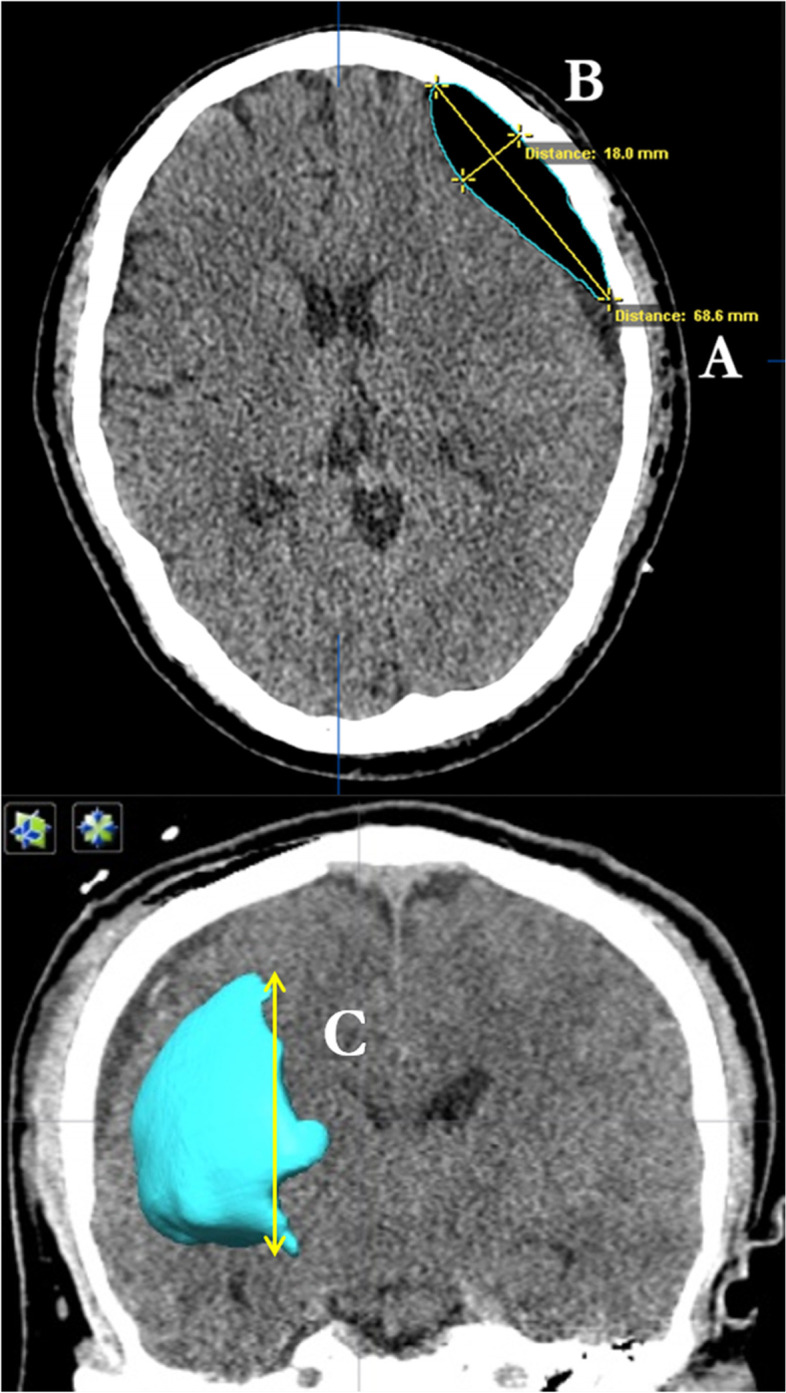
Axial postoperative CT brain scan of cSDH with pneumocephalus. For the ABC/2 method, “A” was defined as the largest longitudinal linear length of the pneumocephalus on the axial plane, “B” was the maximum width from the inner table of the skull to the cerebral cortex perpendicular to A on the same slice (upper), and “C” was the height of the pneumocephalus on a coronal plane, as determined by multiplying the number of CT scan slides with the pneumocephalus visible by the slice thickness (in this study, 5 millimetres (mm) in thickness). The estimated volume was a multiple of A, B, and C and then divided by 2. For the computer-assisted pneumocephalus volumetric measurement, semiautomatic segmentation was traced in each slice for computer-assisted volume calculation (lower) and 3D reconstruction of the pneumocephalus for visualization
